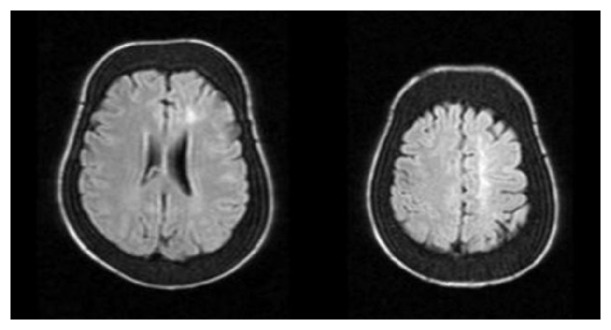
Magnetic resonance imaging in patient with thalassemia major showed flair signal intensity abnormalities in left watershed area and diploe space expansion.
